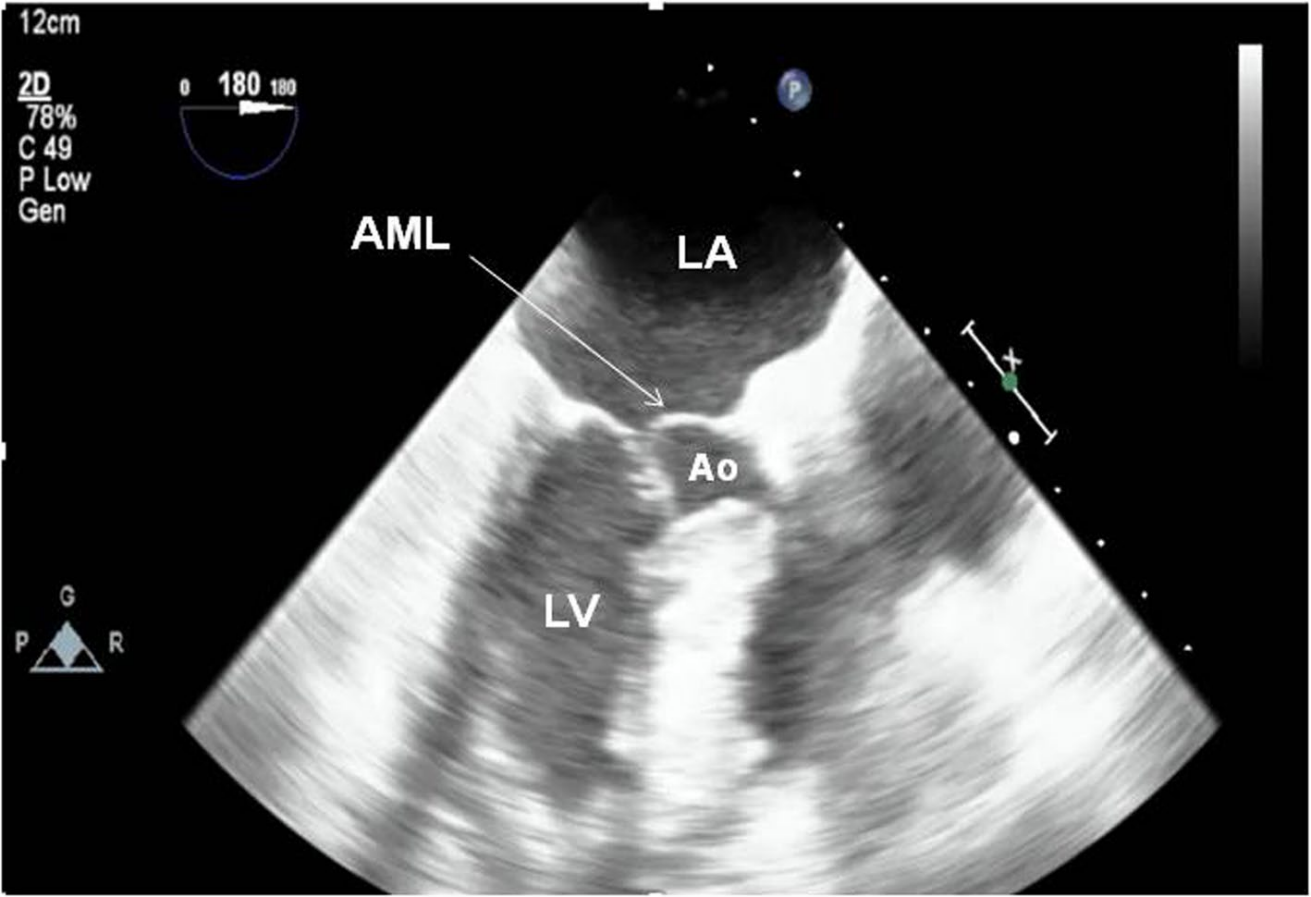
Postoperative transesophageal echocardiography in the intensive care unit (midesophageal long axis view) revealed systolic anterior motion of the anterior mitral leaflet into the left ventricular outflow tract. LA = left atrium; LV = left ventricle; Ao = aorta; AML = anterior mitral leaflet. An additional movie file shows this in more detail (see Additional file 2)
